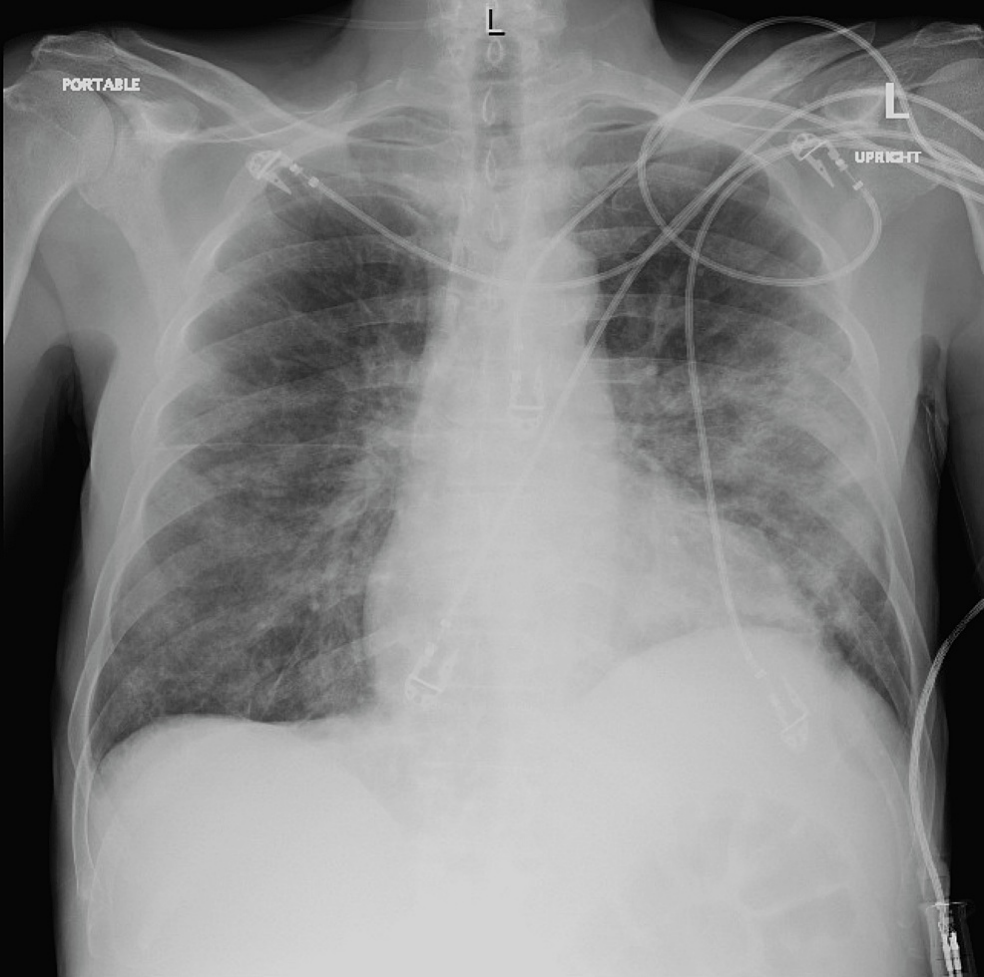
Chest X-ray on the day of admission
